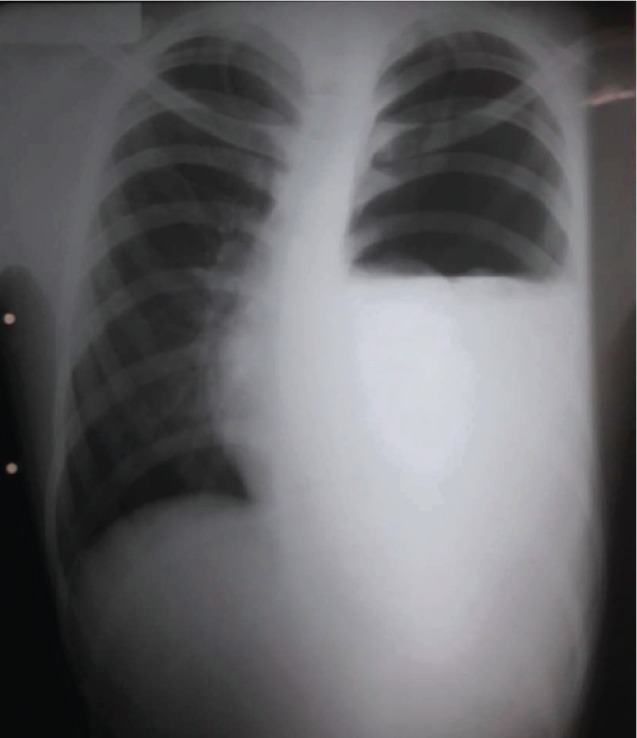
Chest X-ray revealed left pneumothorax with niveau level.
